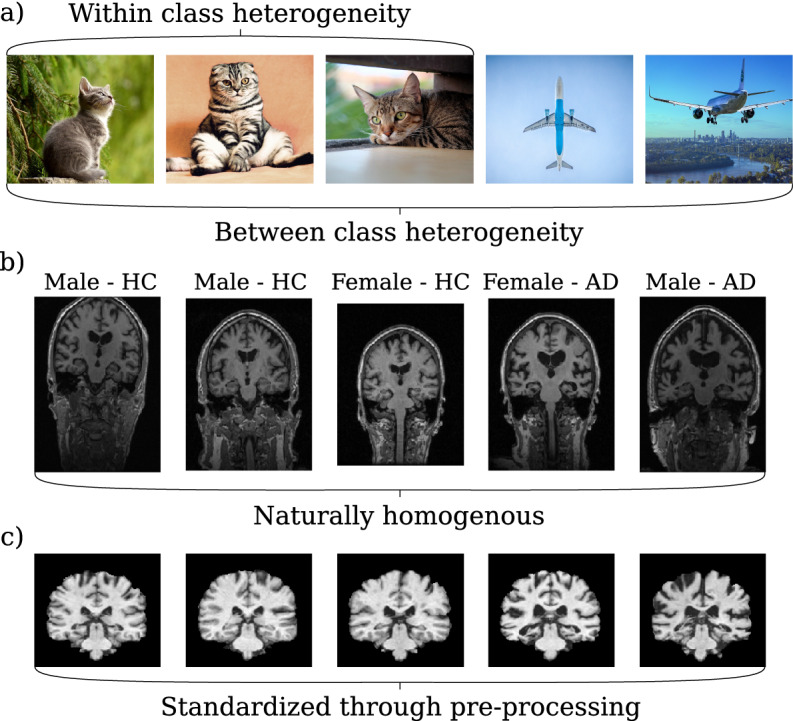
(a) Natural images are typically heterogeneous both within and between classes. (b) MR images of the human brain have homogeneous structures even among different sexes and between healthy subjects (HC) and diseased subjects (AD). (c) Through sophisticated pre-processing techniques, MR images are standardized to a common template reducing their variance further.
